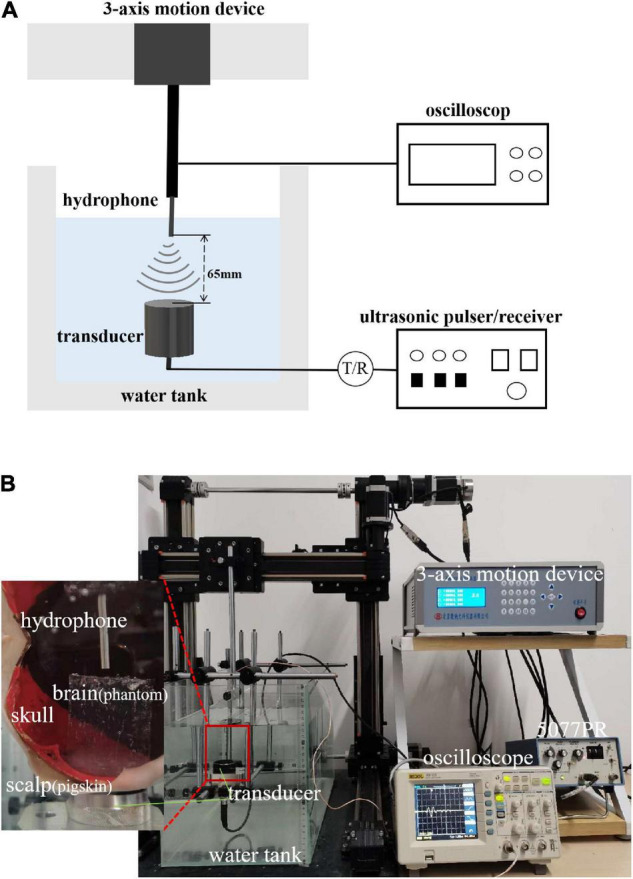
(A) Layout of experiment equipment. The experimental system consisted of a water tank, 3-axis motion device, hydrophone, ultrasound transducer, ultrasonic pulser/receiver, and oscilloscope. (B) Scene of experiment. The figure shows the equipment and its corresponding position. The relation of the equipment to the position of the samples (scalp, skull bones, and brain tissue) is also shown.
